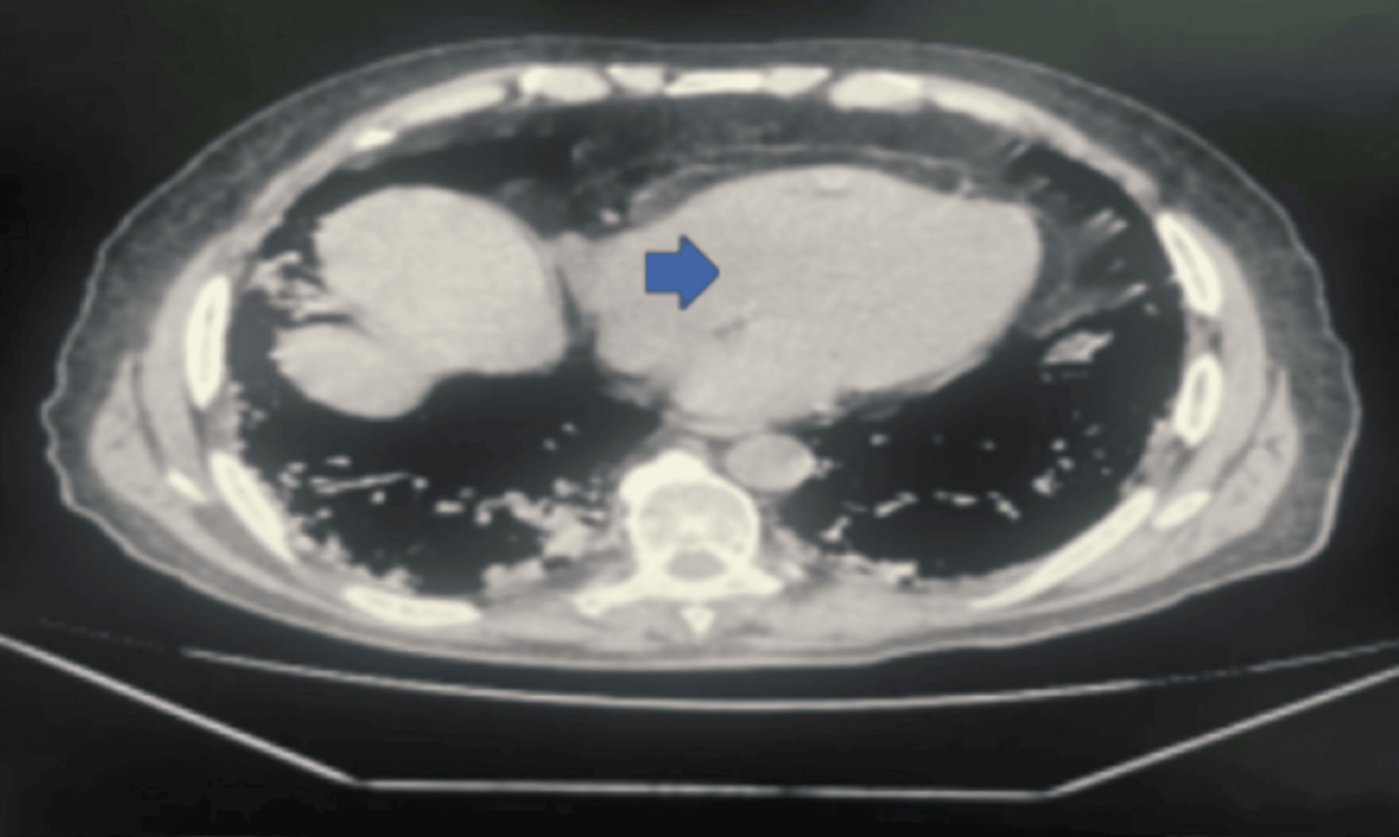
Axial CT scan showing a left paramedian retroperitoneal mass measuring 80 × 76 mm with locoregional invasion CT: computed tomography
